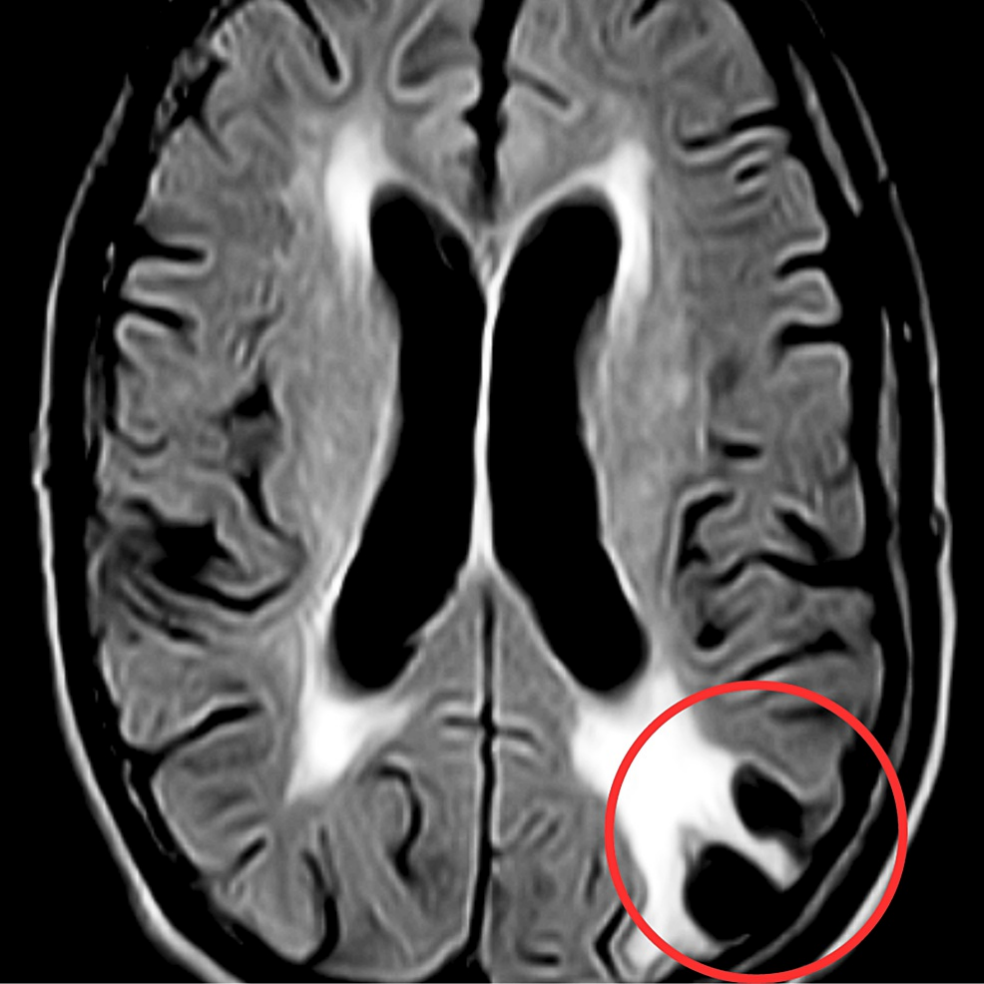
Magnetic resonance imaging of the brain The red circle shows encephalomalacia with surrounding gliosis noted in the left parietal-occipital lobe and ex vacuo dilatation of the occipital horn of the left lateral ventricle.
